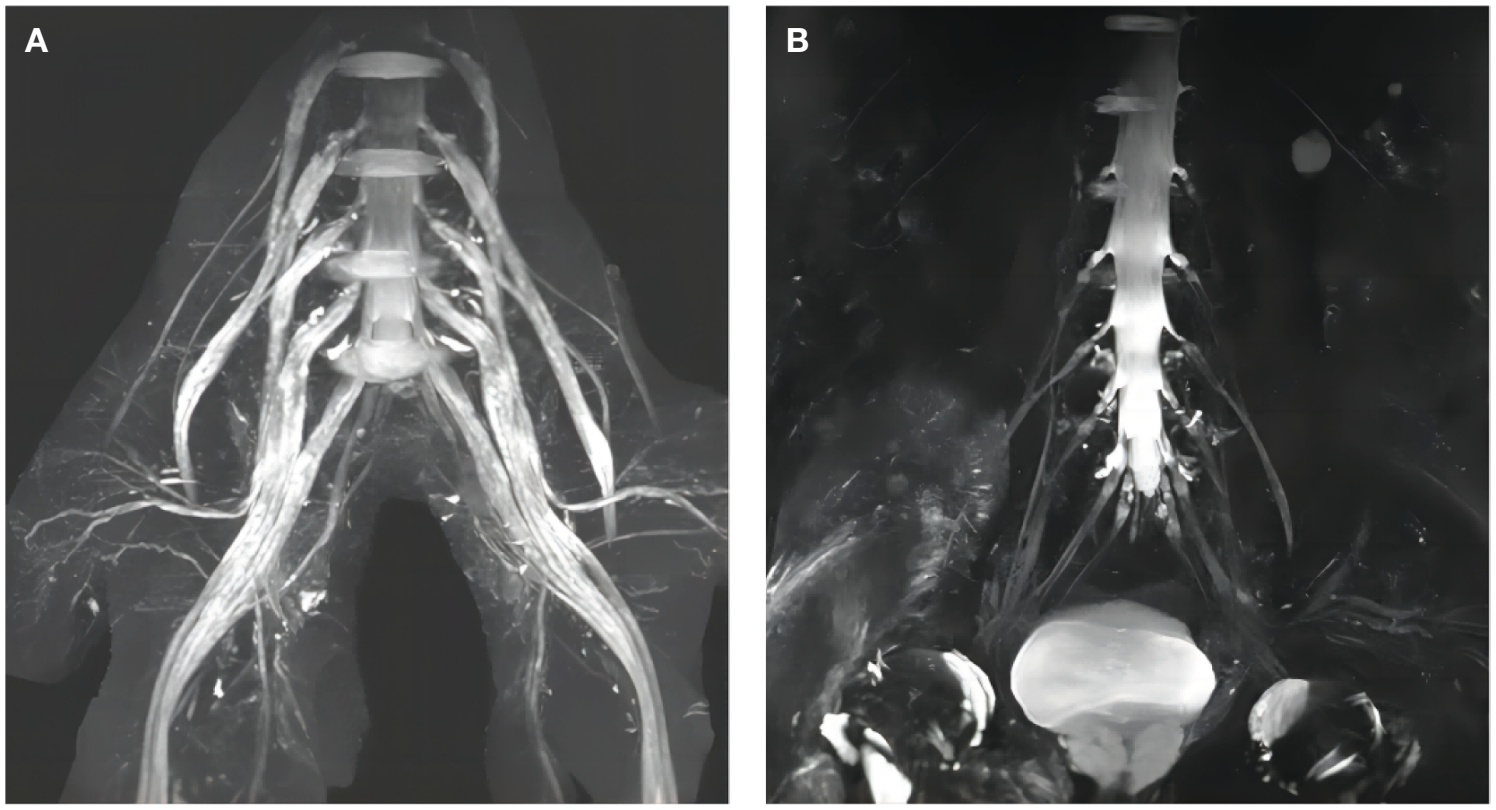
Lumbosacral plexus MRI findings. (A) Diffuse thickening of the lumbosacral nerve roots of a patient with AN with anti-NF155 antibodies; (B) Normal lumbosacral nerve roots of a serologically negative patient with CIDP.
